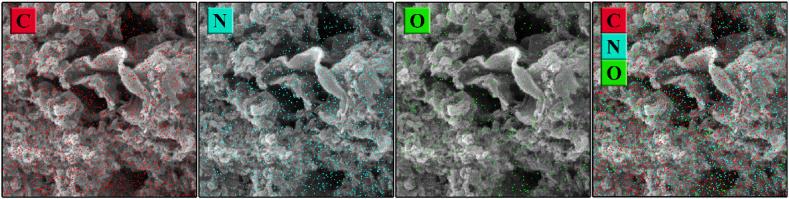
Energy-dispersive X-ray elemental mapping of C55 sample.
